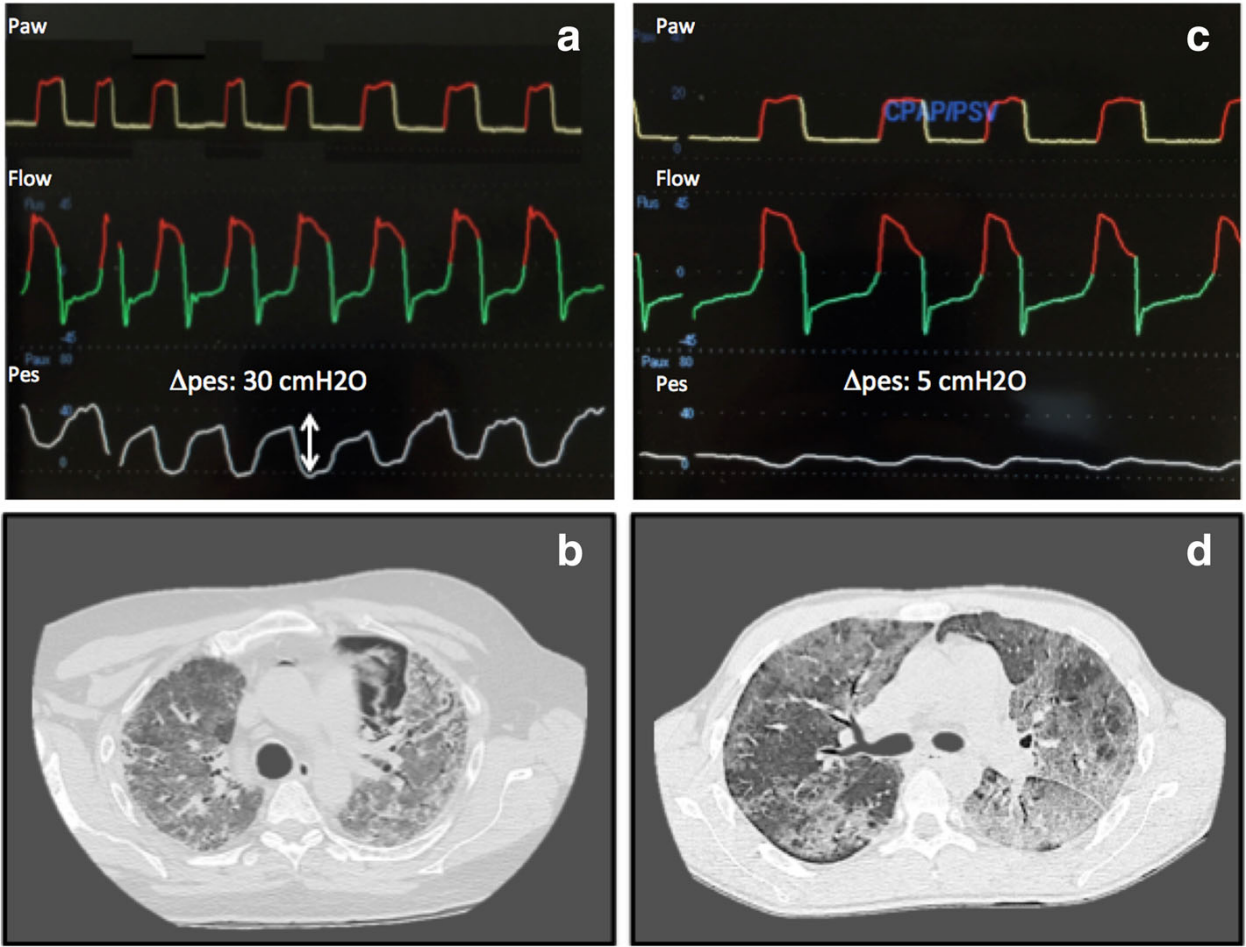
a Patient with AE-IPF during assisted spontaneous breathing with end-expiratory positive pressure of 4 cmH_2_O and pressure support of 10 cmH_2_O. Note ΔPes of 30 cmH_2_O due to respiratory drive hyperactivity. b Thorax CT scan performed on same patient as (a), showing anterior left pneumothorax probably due to high transpulmonary pressure. Note homogeneous increase of parenchymal density. c Patient with AE-IPF during assisted spontaneous breathing with end-expiratory positive pressure of 4 cmH_2_O and pressure support of 10 cmH_2_O. Note ΔPes of 5 cmH_2_O due to normal activation of respiratory drive. d Thorax CT scan performed on same patient as (b) showing nonhomogeneous opacities in lung parenchyma. Pes esophageal pressure. Paw airway pressure
